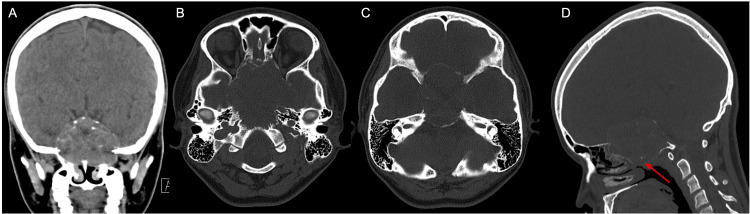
(A) CT images in coronal plane with soft tissue window show a slightly hyperdense soft tissue mass. (B) In the axial plane with bone window more inferiorly shows a lytic expansile mass with scalloping of the clivus and (C) more superiorly shows thinning of the bone without frank destruction. (D) The sagittal plane with bone window demonstrates the expansile lytic mass centered at the clivus containing hyperdense punctate foci likely representing punctate calcifications or residual bone (red arrow).
